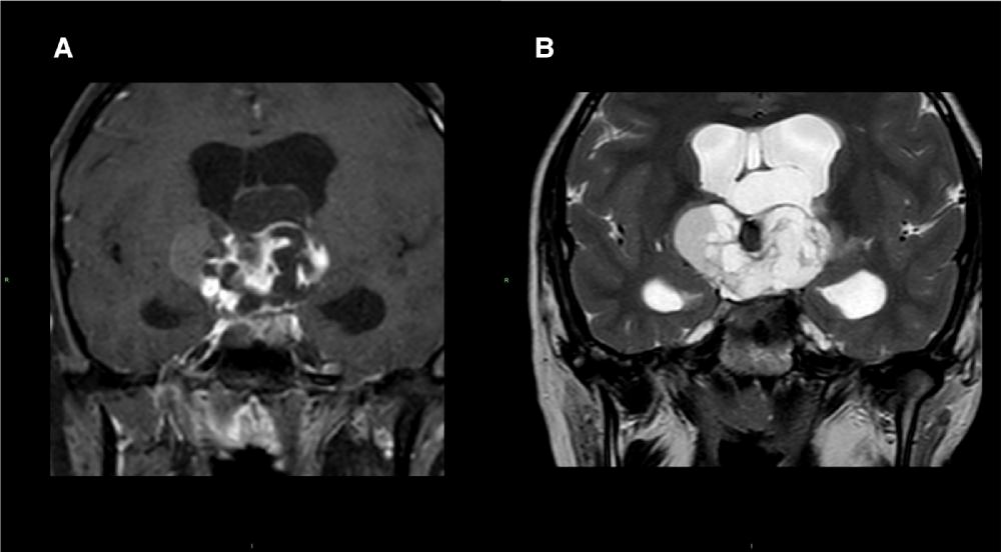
(a) and (b): Coronal T2 and enhanced T1-weighted images [figures (a) and (b), respectively] demonstrate a large expansive formation (5 × 4 cm) with irregular enhancement and associated cystic components in the median suprasellar region, with expansion to the third ventricle imprinting the chiasma region, obliterating the interventricular foramina of monroe, with dilatation of the ventricular system in the supratentorial site and caudally to the prepontine cistern (not shown). The lesion is iso-hyperintense in T1-weighted and hyperintense in T2-weighted images.
